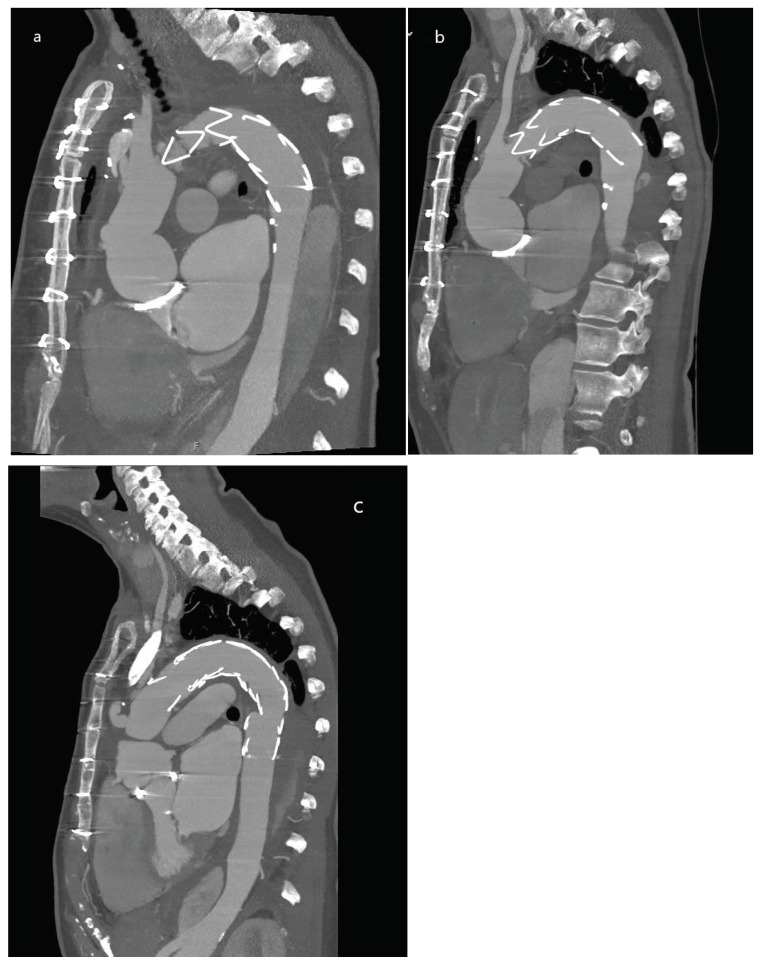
Computed tomography images of patient with aortic arch aneurysm treated with FET and subsequent TEVAR extension to address distal bird beak. Patient with aortic arch aneurysm treated with FET (a) who subsequently experienced increase in distal angle of FET prothesis, leading to a distal bird beak (b), which was addressed with TEVAR extension (c).
